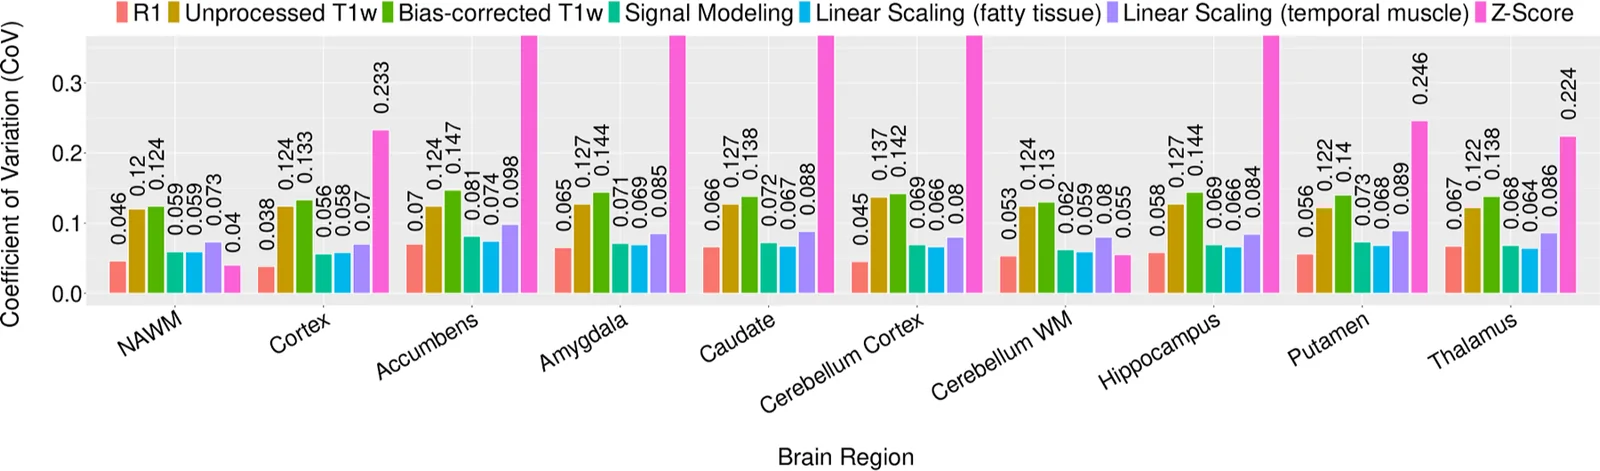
Inter-image variance in brain regions. The inter-image intensity variance is presented for each brain region and image type in terms of CoV. Linear scaling with fatty tissue and signal modeling consistently yields CoVs similar to R1 maps. Linear scaling with temporal muscle results in slightly larger CoVs; Z-score standardization provides the lowest CoVs in WM but the largest CoVs in all other regions (CoVs in the Accumbens, Amygdala, Caudate, Cerebellum Cortex, and Hippocampus are not completely displayed, and range between CoV = 0.6 and CoV = 3.2). Abbreviations: CoV: coefficient of variation, NAWM: normal-appearing white matter, T1w: T1-weighted, WM: white matter.
